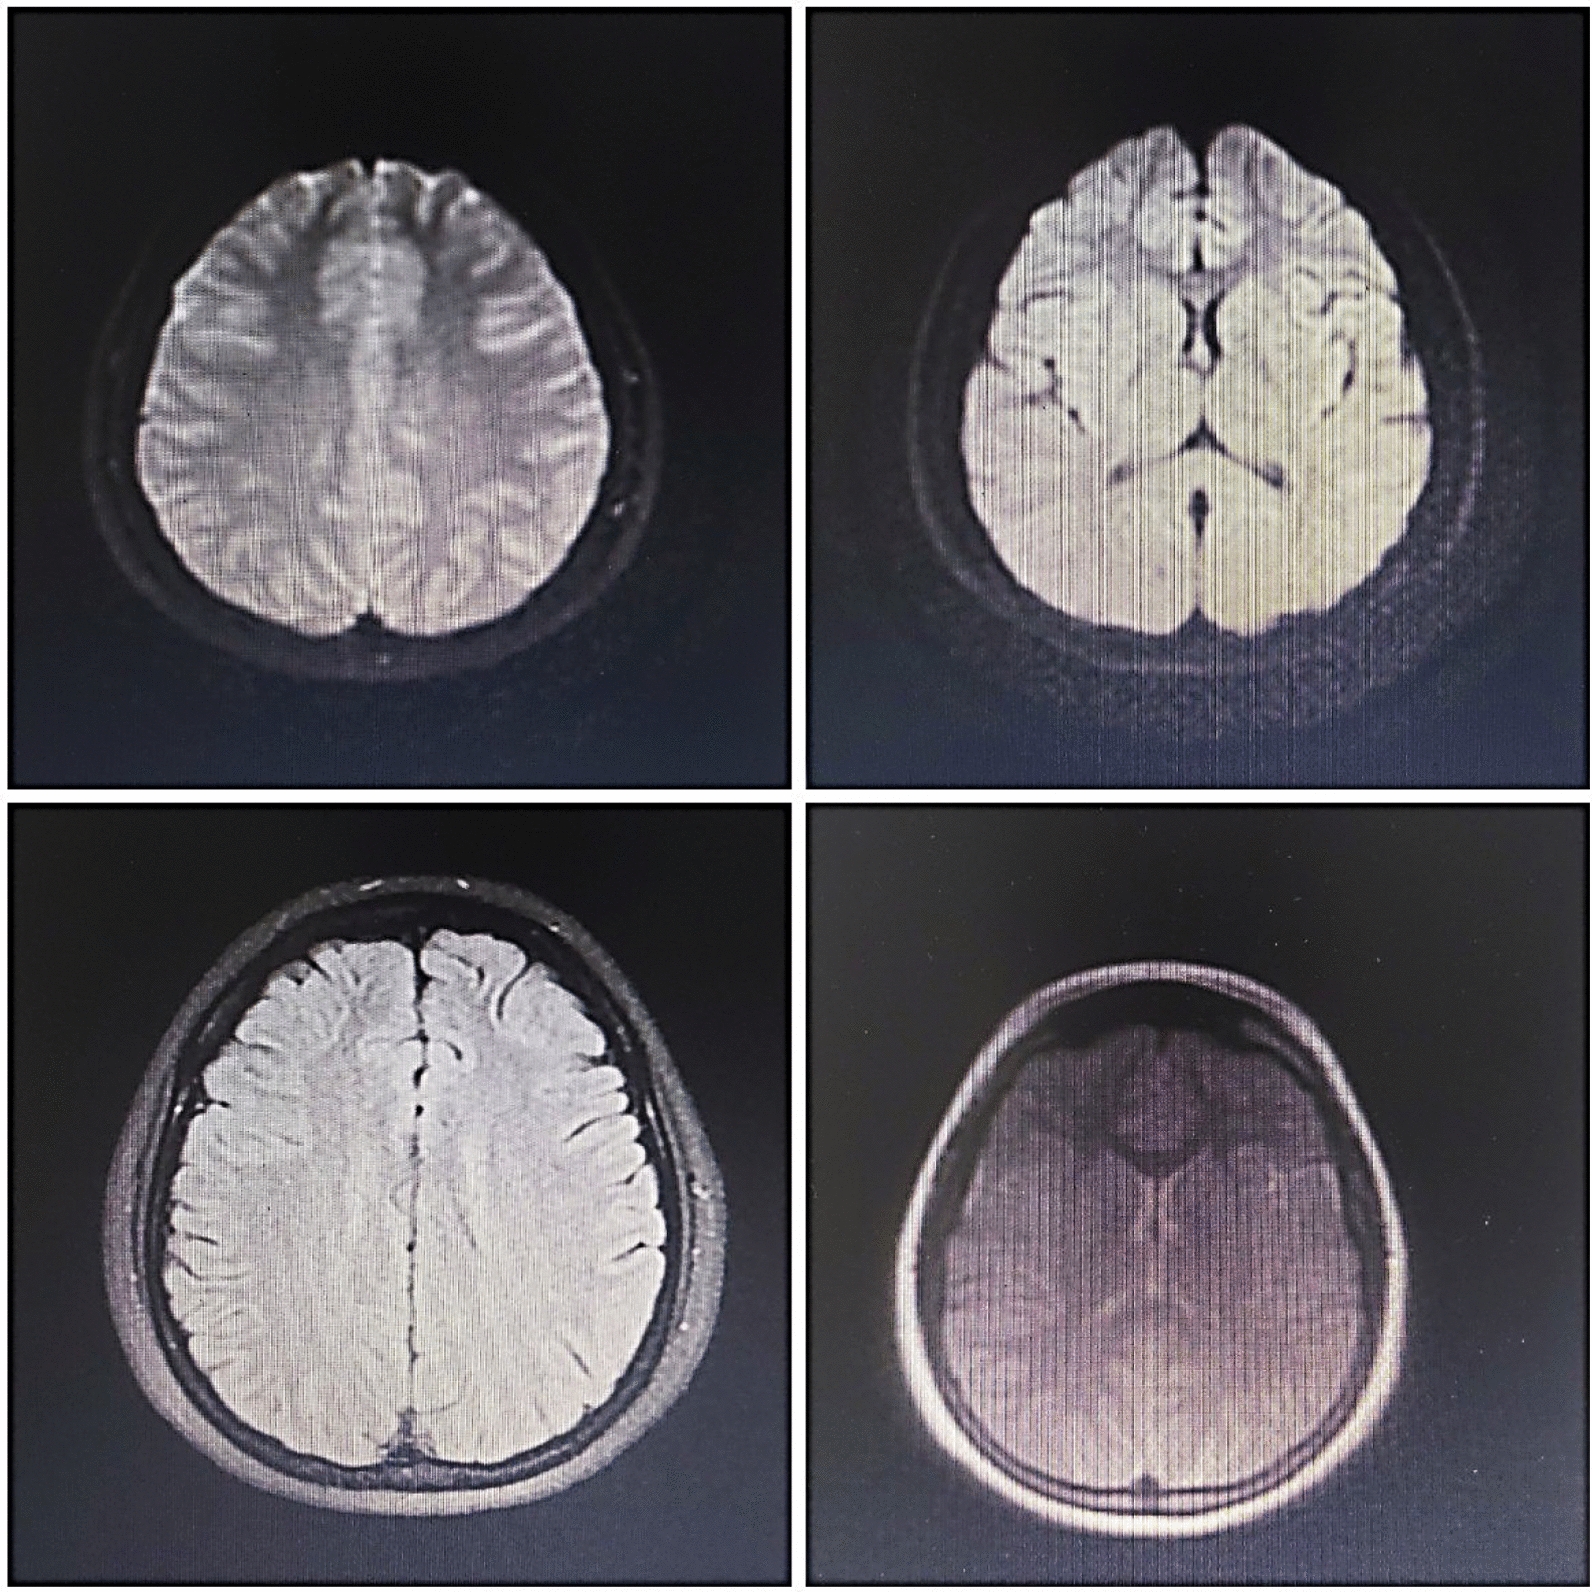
Magnetic resonance imaging findings. Brain magnetic resonance imaging sequences revealed no suspicious signal alterations a the time of diagnosis
